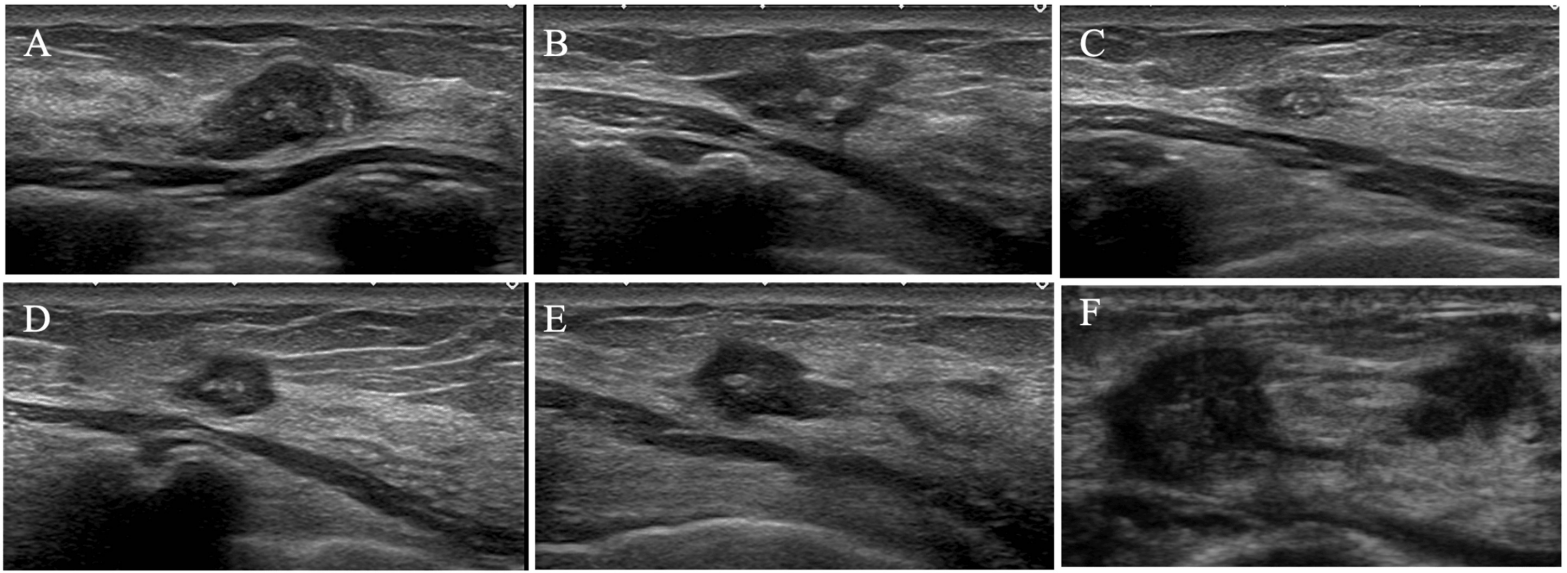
Ultrasound findings Ultrasound of the florid adenosis nodule showed continued shrinkage (A: 60 months, B: 72 months, and C: 78 months) after the vacuum-assisted biopsy, enlargement of the nodule from the 90th month (D) onwards (E: 114 months), and invasive carcinoma findings at 120 months (F).
